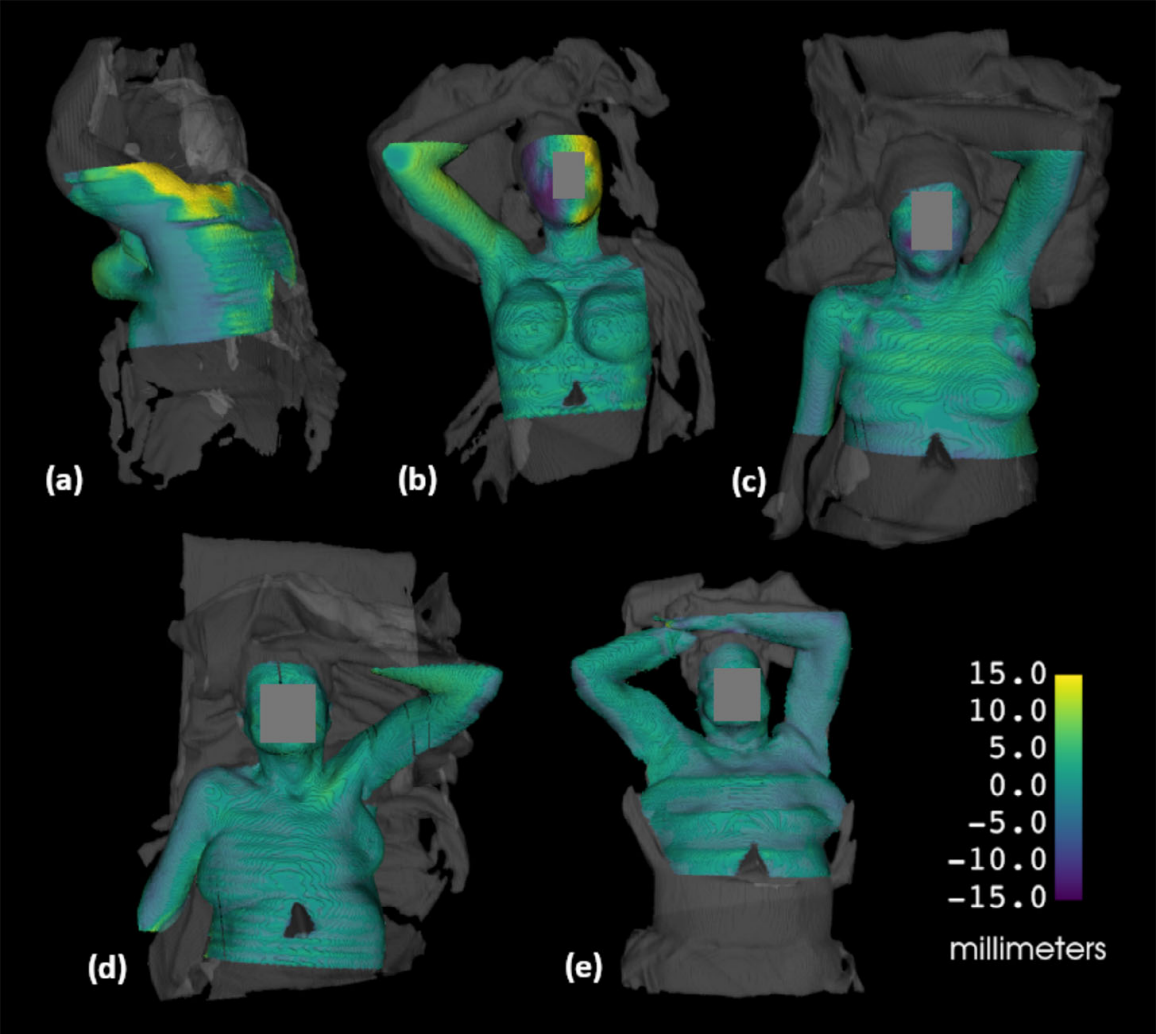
Model to model distance maps for the 2 mm CT rind to relevant 3D surface FOV, overlayed with the full 3D surface in grey. (a) Patient 1, (b) Patient 2, (c) Patient 3, (d) Patient 4, (e) Patient 5.
